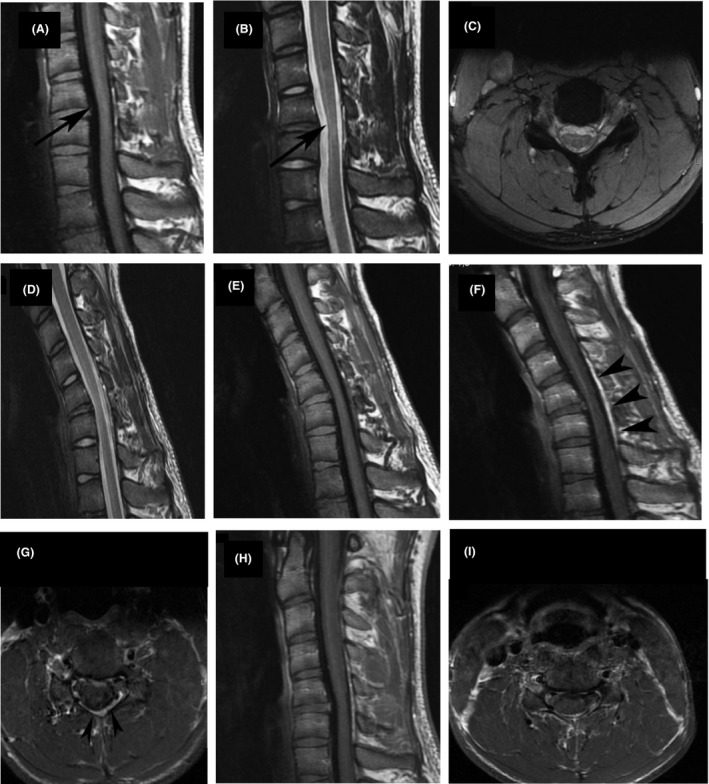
Neutral position MRI of the spine, sagittal T1‐weighted image (A) and T2‐weighted image (B) showing straightening of the cervical curvature and localized cord atrophy at C5‐C6 level (arrows).On axial T2‐weighted image (C) spinal cord shows normal signal intensity. Flexion sagittal T2‐weighted image (D) and T1‐weighted (E) showing anterior displacement of dorsal dura and a crescent epidural compartment which shows enhancement on postcontrast sagittal (F) and axial (G) T1‐weighted images (arrowheads). Repeated acquisition on neutral position shows elimination of enhancement on sagittal (H) and axial (I) postcontrast T1‐weighted images.
